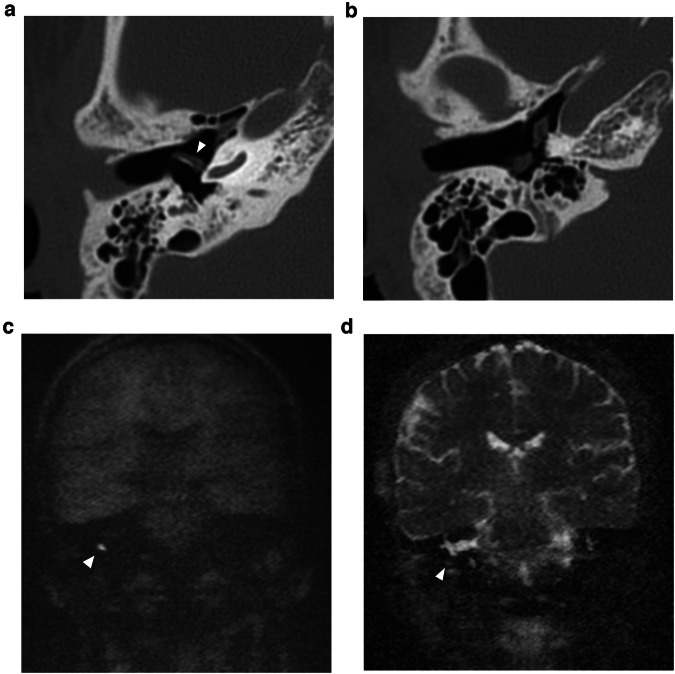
Sixteen-year-old male patient with conductive hearing loss on the right. a, b CBCT showing an irregular and discontinuous soft tissue lesion growing along the malleus handle and in the mesotympanum. Diffusion restriction on the b1000 DWI (c) and corresponding ADC map (d), in keeping with a CC. The growth pattern is suggestive of an “open-type” morphology
